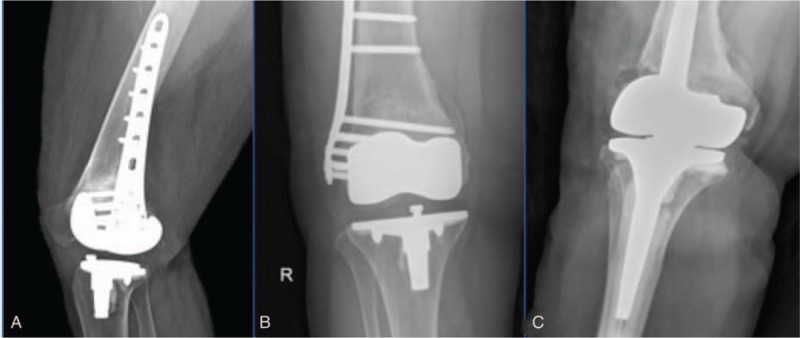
Anteroposterior and lateral radiographs of patient 2, the LISS plate was not centered on the femoral shaft (A), the gap of the lateral compartment was wider under varus stress (B), placement of the prosthesis was appropriate (C).
